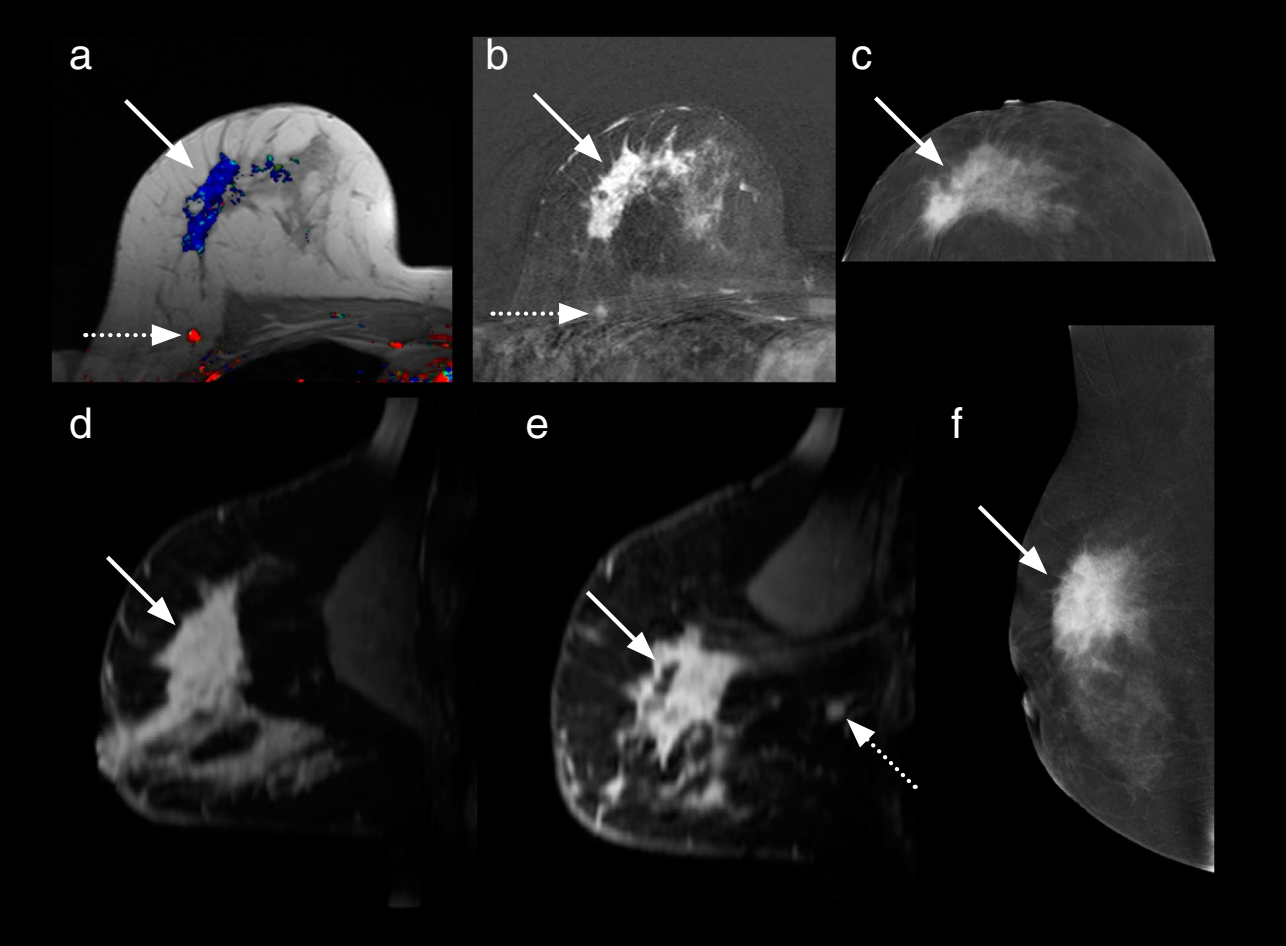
Case number 5 of Table 3. 5. A 47-year-old patient with a palpable right breast mass. Images of the right breast: MRI (a) axial post-contrast T1-weighted non-fat suppressed image with colour overlay, (b) Fat suppressed axial post-contrast (d, e) sagittal post-contrast T1-weighted subtraction images. CEM (c) recombined CC view, orientated to match the axial MRI images (f) recombined MLO view. CEM and MRI both demonstrated the index lesion (solid arrows), a pleomorphic grade 3 invasive lobular carcinoma. MRI showed a moderately enhancing additional 6-mm mass in the postero-superior aspect of the right breast (dotted arrows). This lesion is out of the field of view on CEM. The additional lesion was a metastatic intramammary lymph node. *CEM* Contrast-enhanced mammography, *MRI* Magnetic resonance imaging, *CC* Cranio-caudal, *MLO *Mediolateral oblique
